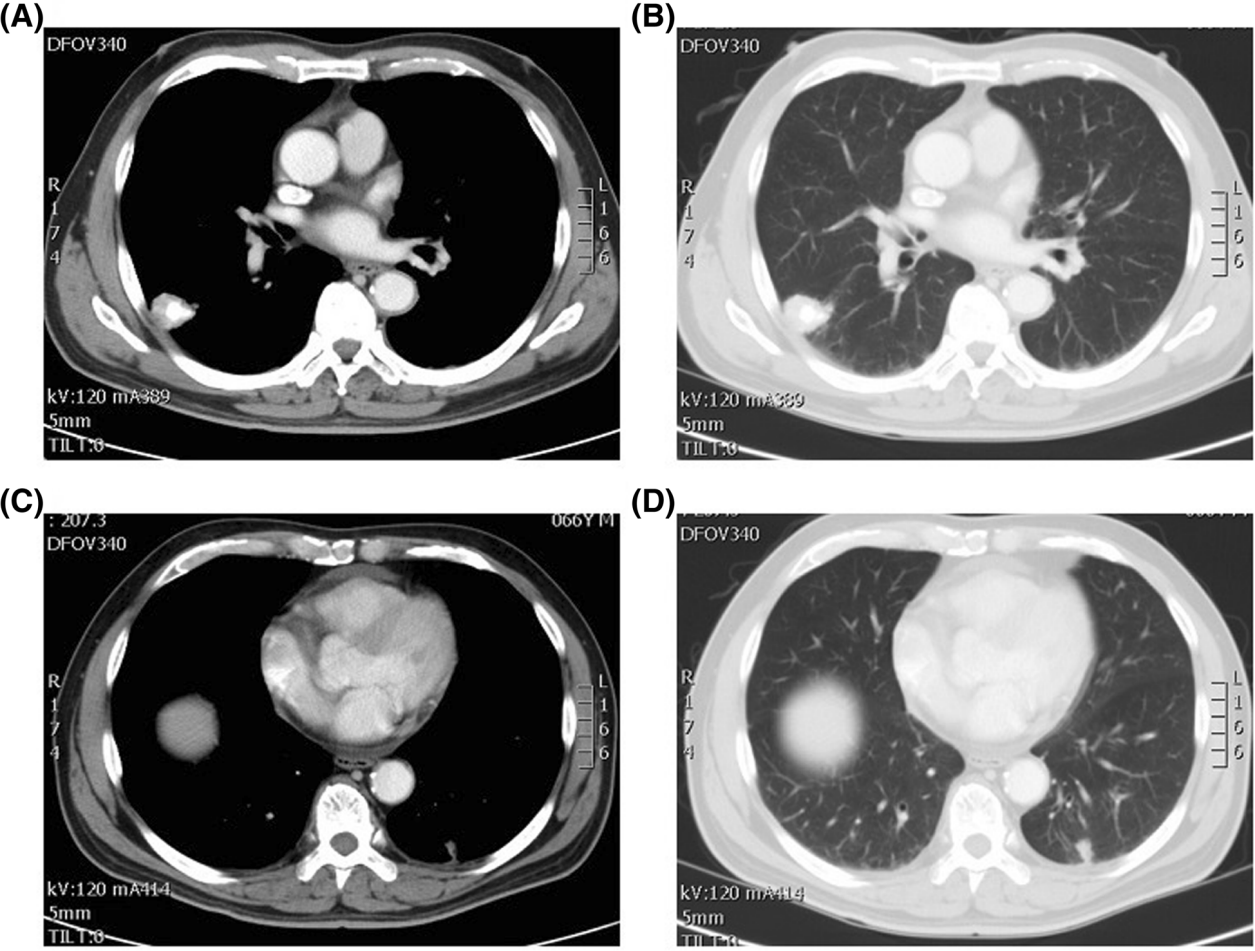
Synchronous multiple primary lung cancer of the 68-year-old patient. Chest computed tomography showing a right lower lobe mass (axial view), b right lower lobe mass (lung window view), c left lower lobe mass (axial view), d left lower lobe mass (lung window view)
